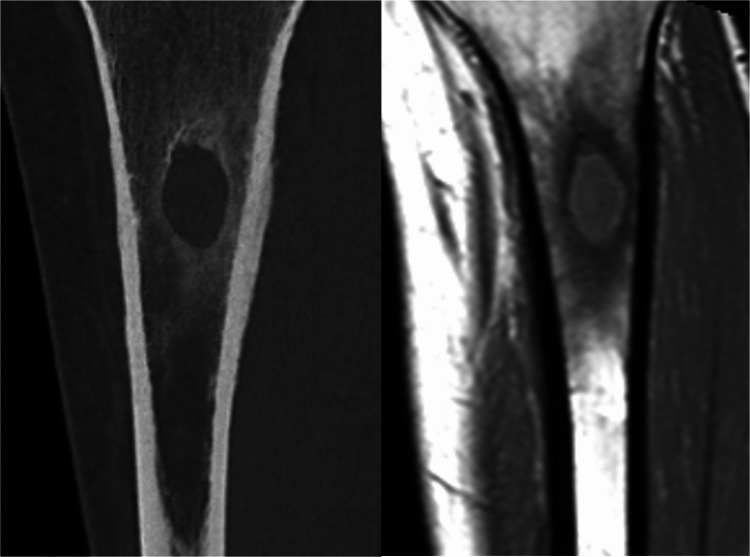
Thirty-eight-year-old male with Brodie’s abscess. Coronal CT reconstruction (left) shows a well-defined lytic lesion within the proximal tibial metaphysis with a peripheral sclerotic margin, adjacent sclerosis and periosteal new bone formation medially. Corresponding coronal T1-weighted MRI (right) shows a relatively well-defined lesion with central hypointense signal, peripheral hyperintense margin (‘penumbra’ sign indicating granulation tissue) and surrounding oedema-like signal change
